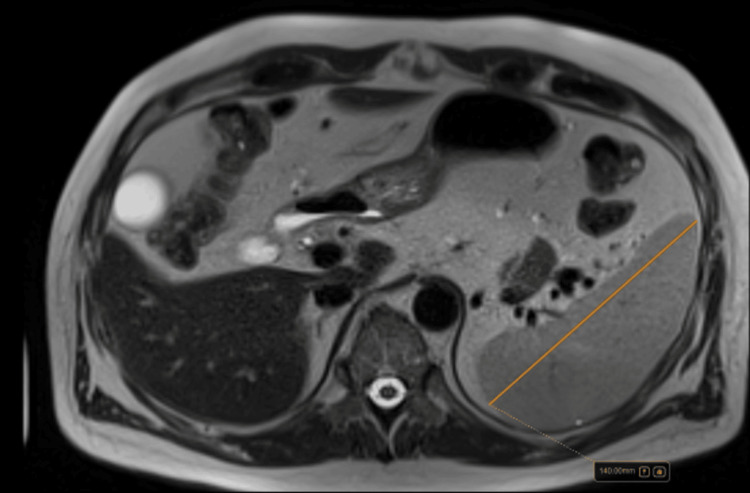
Follow-up MRI (axial T2-weighted image) demonstrating splenomegaly (maximum craniocaudal length ~140 mm)
